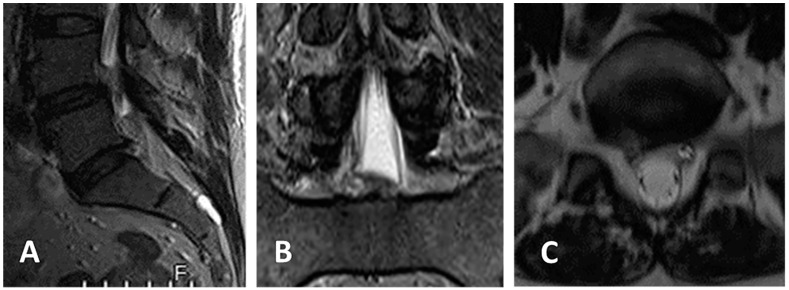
(A–C) Postoperative MRI on the same day of operation shows complete epidural decompression after removal of the herniated disc with the TESSYS technique. VAS 1, ODI 6.
